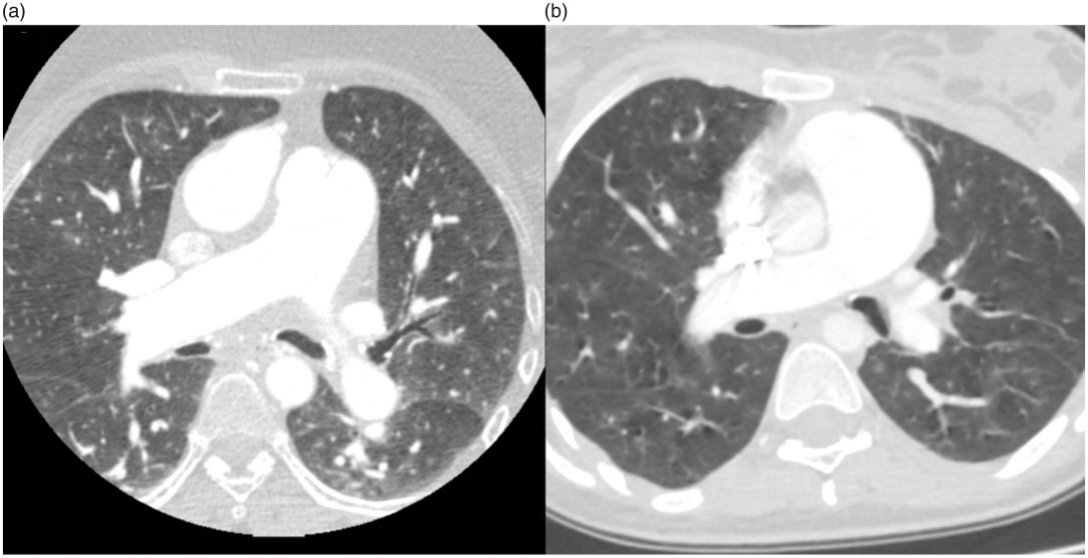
Chest CT axial slices at the level of the main right and left bronchi
